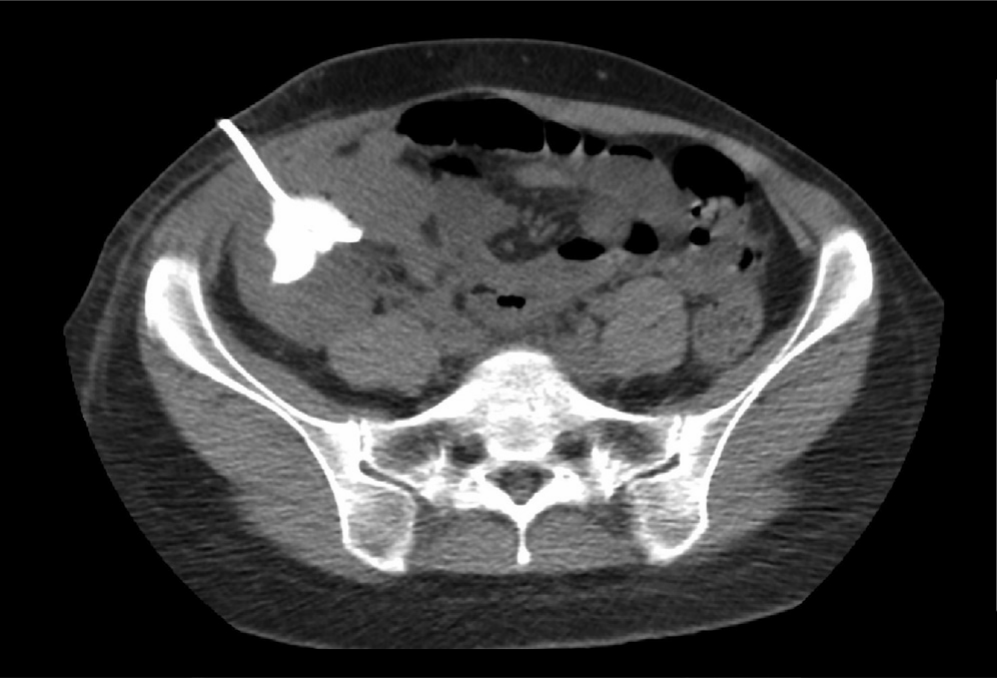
Contrast-enhanced computed tomography study showing no connection between cyst and urinary tract.
